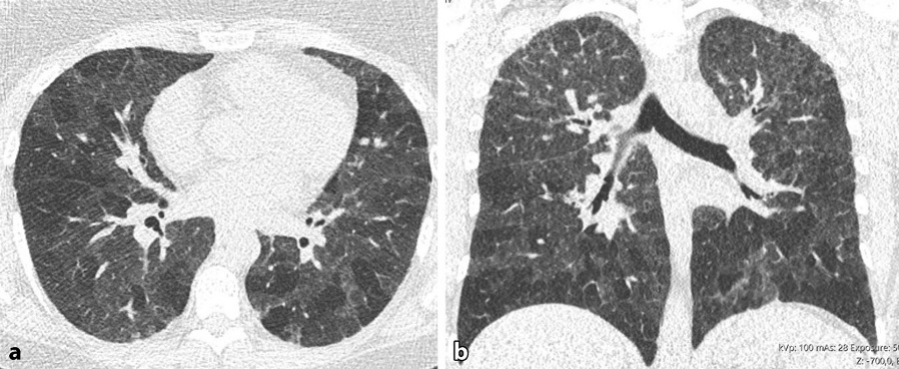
Inflammatorische exogen-allergischen Alveolitis (EAA). a Axiale und b koronare multiplanare Reformation (MPR) einer nativen High-Resolution-Computertomographie (HRCT). Diffus in der Lunge verteilt zeigt sich eine Kombination aus Milchglas und Air-Trapping. Zeichen einer Fibrose fehlen
